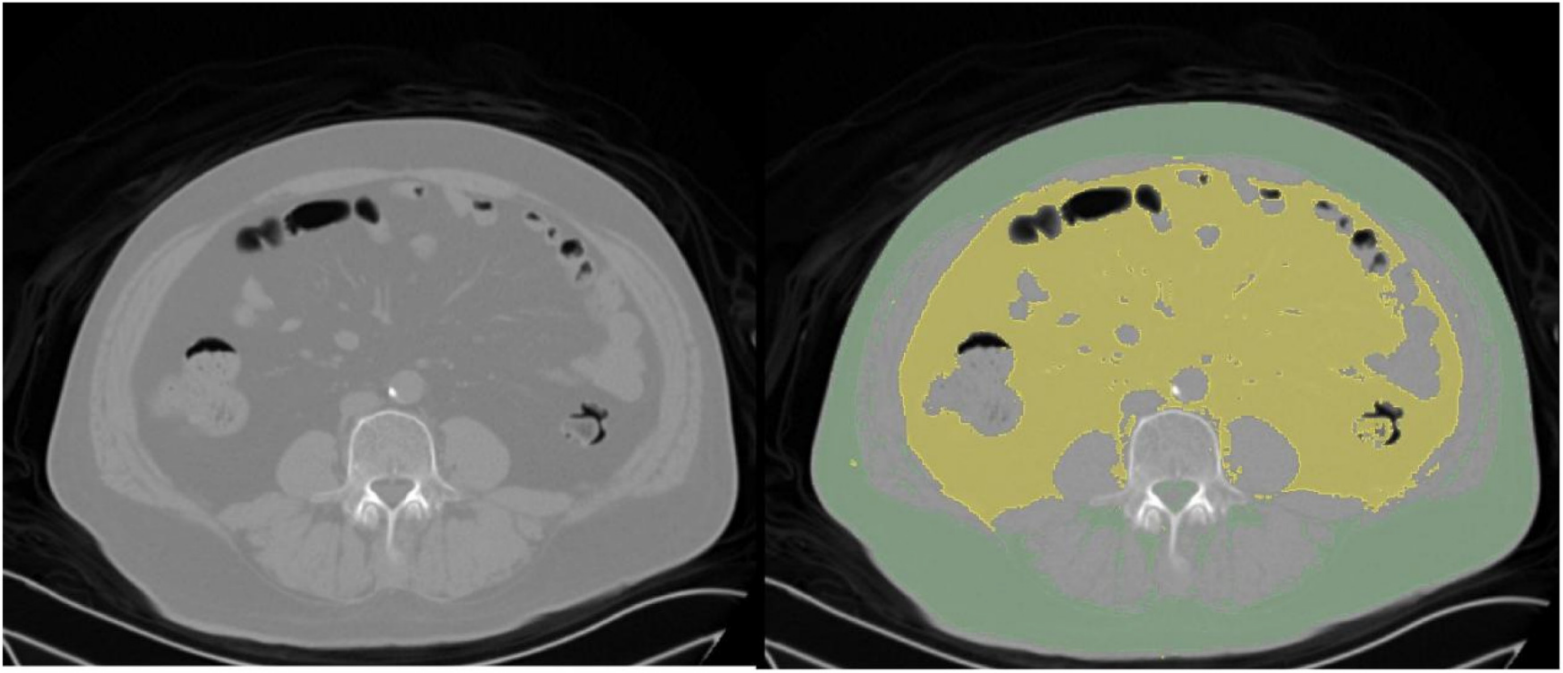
CT scan images from a 60-year-old male patient with a L5 vertebral slip. Left panel: Original CT image displaying the abdominal cross-section. Right panel: Processed CT image with subcutaneous fat highlighted in green and visceral fat in yellow.
